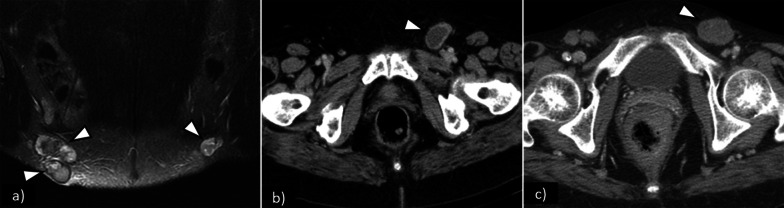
Pathologically proven inguinofemoral lymph node metastasis in different patients (FIGO III): a coronal fat saturation T2WI shows bilateral enlarged heterogeneous lymph nodes with necrotic changes depicted by intra-nodal high-signal intensity areas; b axial CT shows left inguinofemoral enlarged node with low-attenuation necrotic centre; c axial CT shows heterogeneous enlarged left inguinofemoral node
